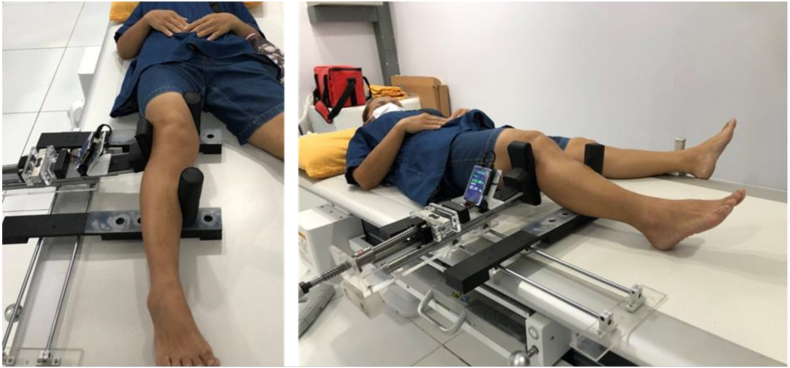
The patient was supine, the knee joint was in a 20° flexion position, the stress device applied a valgus force of 110 N, and an anterior-posterior (AP) knee joint X-ray was taken.
